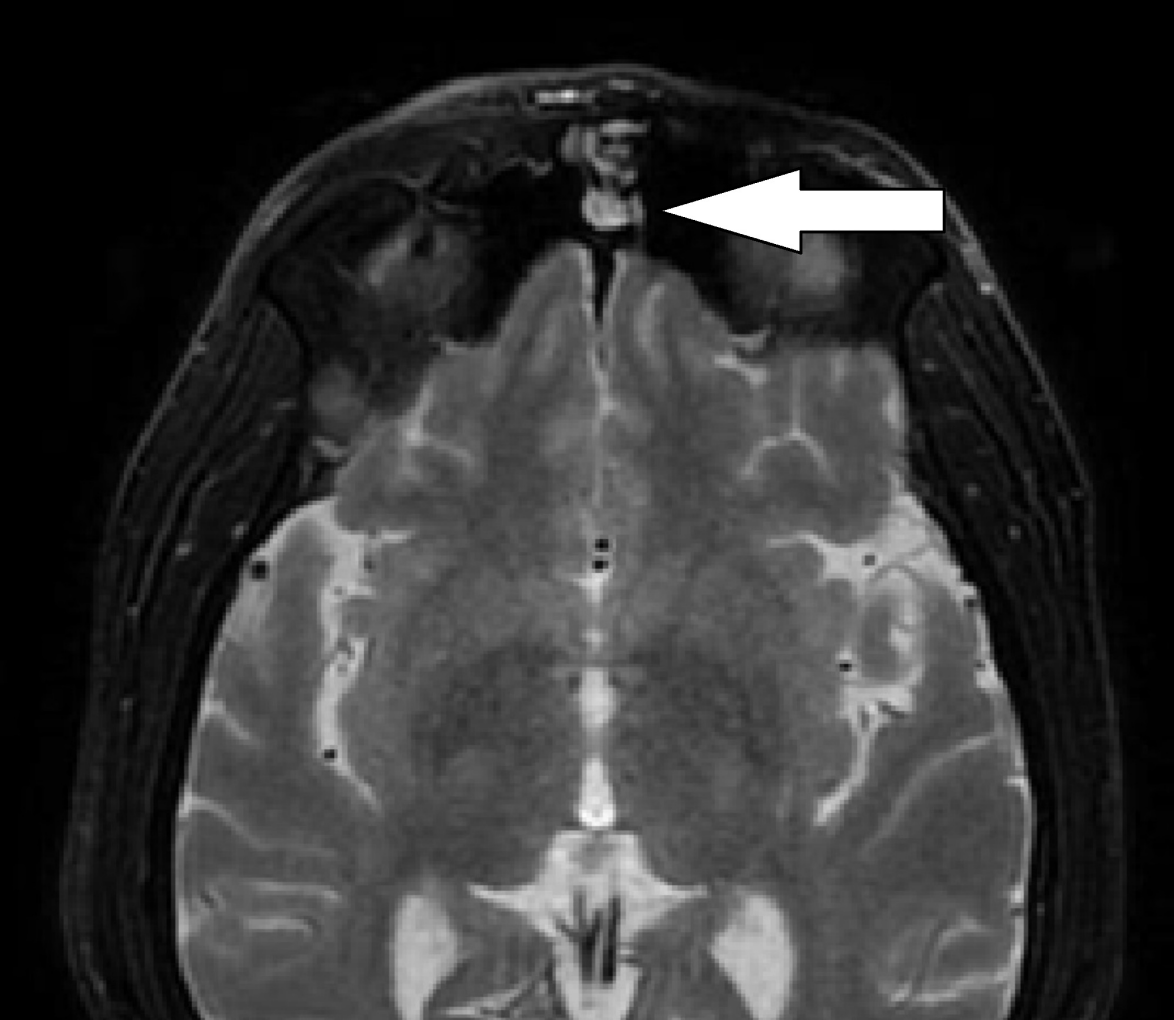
MRI T2-Weighted with Contrast Evidence of a 7 mm mass protruding in the left frontal sinus MRI: magnetic resonance imaging
